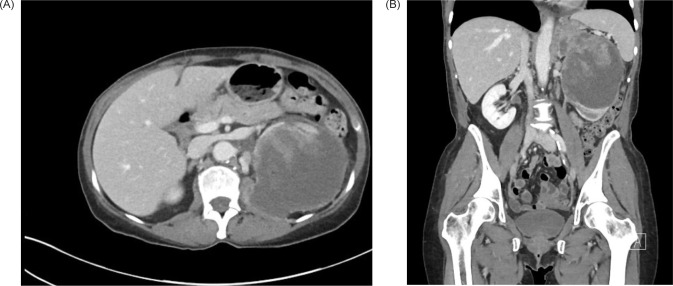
CT of the abdomen and pelvis with IV contrast. (A) Transverse and (B) coronal views demonstrating a 9.2 × 9.3 × 12.4 cm left renal mass with extension into the left psoas.
